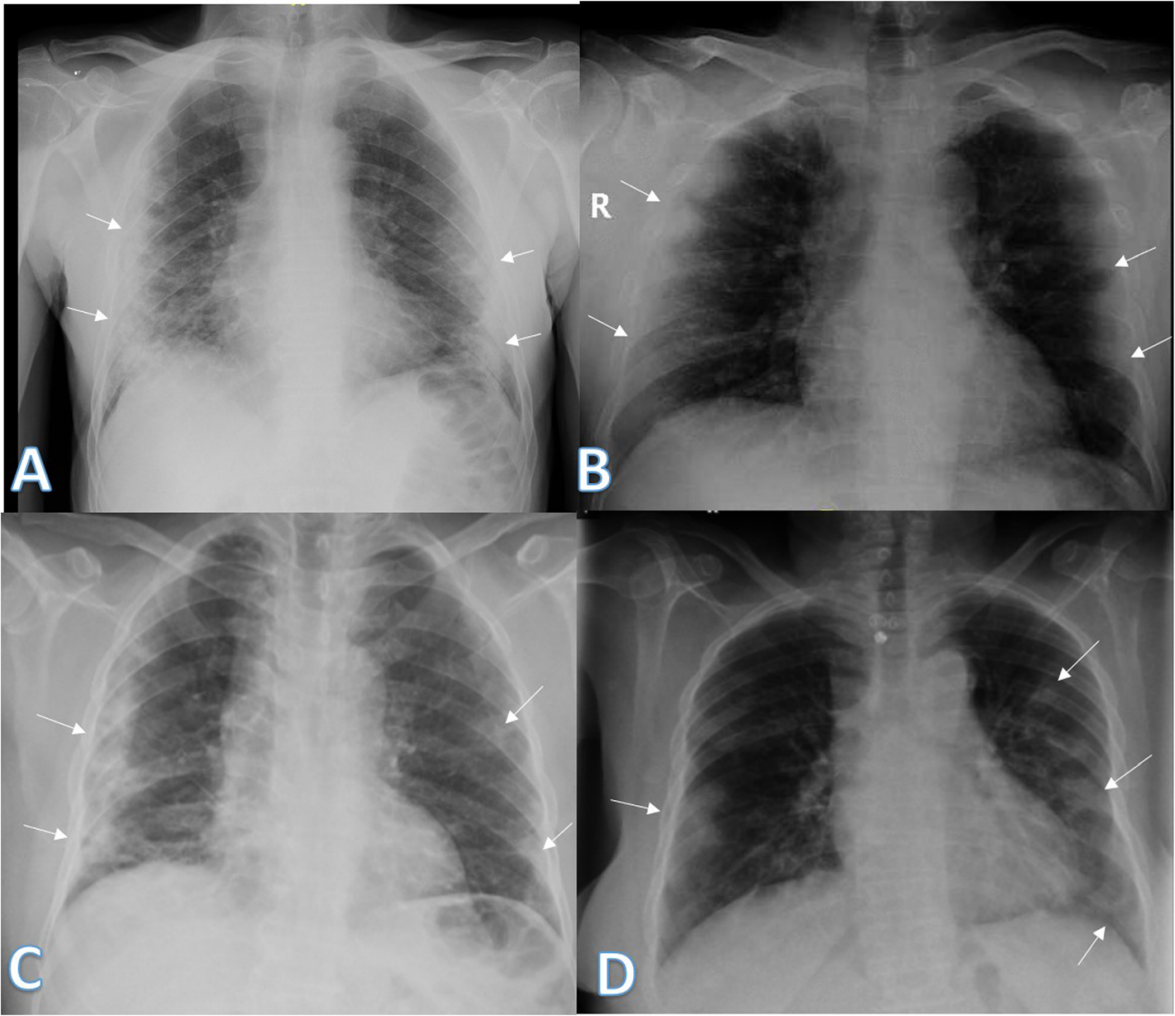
Four different patients with positive COVID-19. a 72-year male was complaining of fever and cough. Initial chest X-ray showed bilateral peripheral mid and lower zonal air space consolidation opacities (arrows). The severity score was 2 for each lung, so TSS was 4. b 44-year male was complaining of SOB, cough, and fever. Initial chest X-ray showed bilateral peripheral zonal air space consolidation opacities along the periphery of both lungs (arrows). The severity score was 2 for each lung so, TSS was 4. c 63-year male was complaining of cough and fever. Initial chest X-ray showed right peripheral mid and lower zonal air space consolidation opacities (arrows), smaller patches of consolidation is seen on the side (arrows), the severity score for the right lung was 2 and for the left lung was 1, so TSS was 3. d 68-year female patient was complaining of SOB and cough. Initial chest X-ray showed bilateral air space consolidation opacities (arrows) of peripheral midzonal distribution in the right lung and neither peripheral nor perihilar mid and lower zonal distribution in the left lung (arrows). The severity score for the right lung was 1 and for the left lung was 2, so the total severity score was 3
